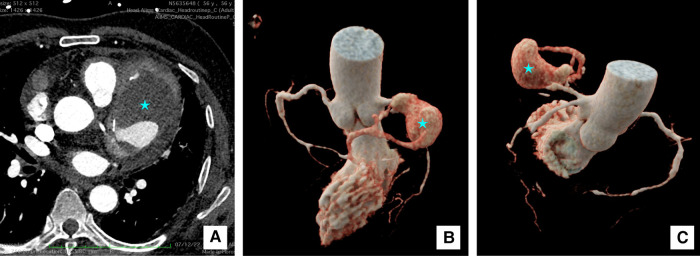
Cardiac computed tomography (CT) images. (*A*) 2D transverse section image at the level of aortic sinus showing giant saccular pseudoaneurysm of left anterior descending artery (*) measuring 8.1 × 5.2 × 4.7 cm with peripheral thrombus and calcific foci; (*B* and *C*) 3D reconstruction images of the pseudoaneurysm (*).
